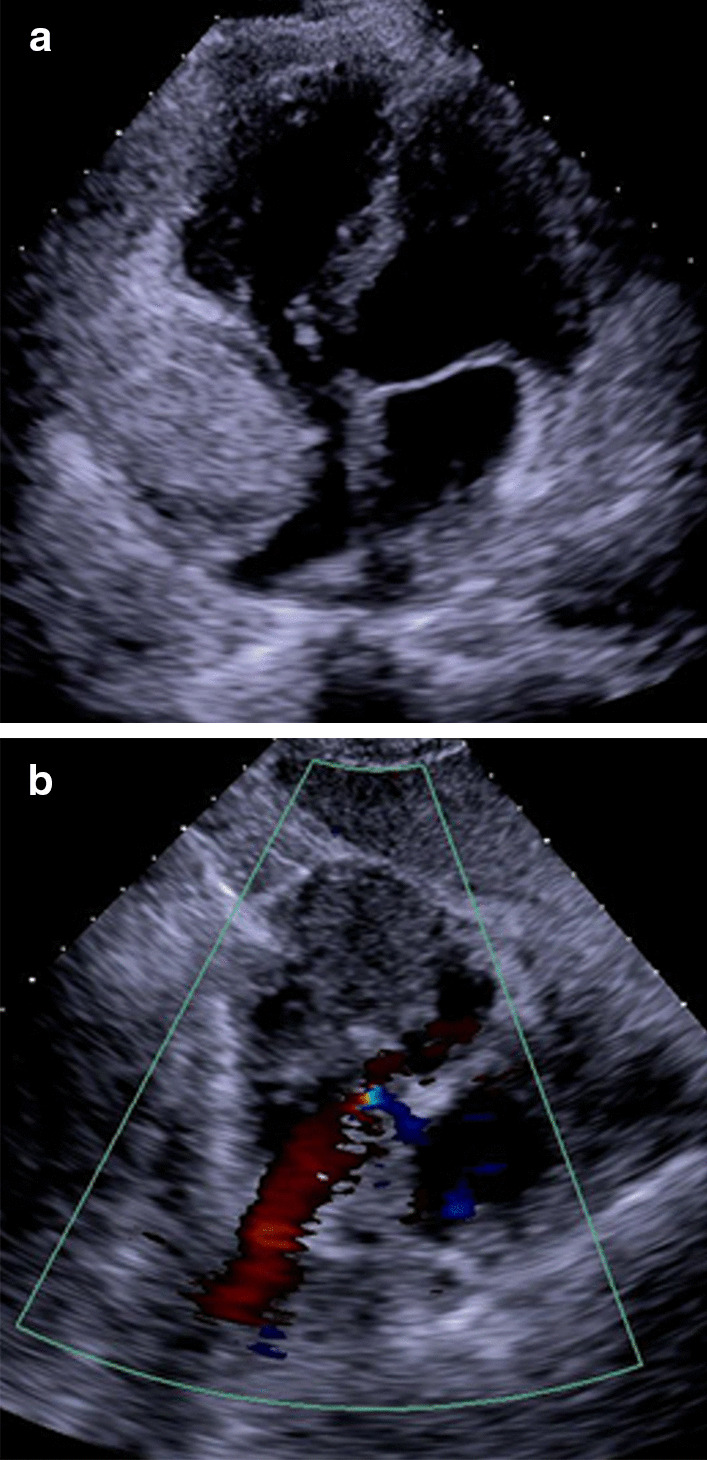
Echocardiography of heart. a The mass arose in the free wall of the right atrium, b indicating intra-neoplastic necrosis
